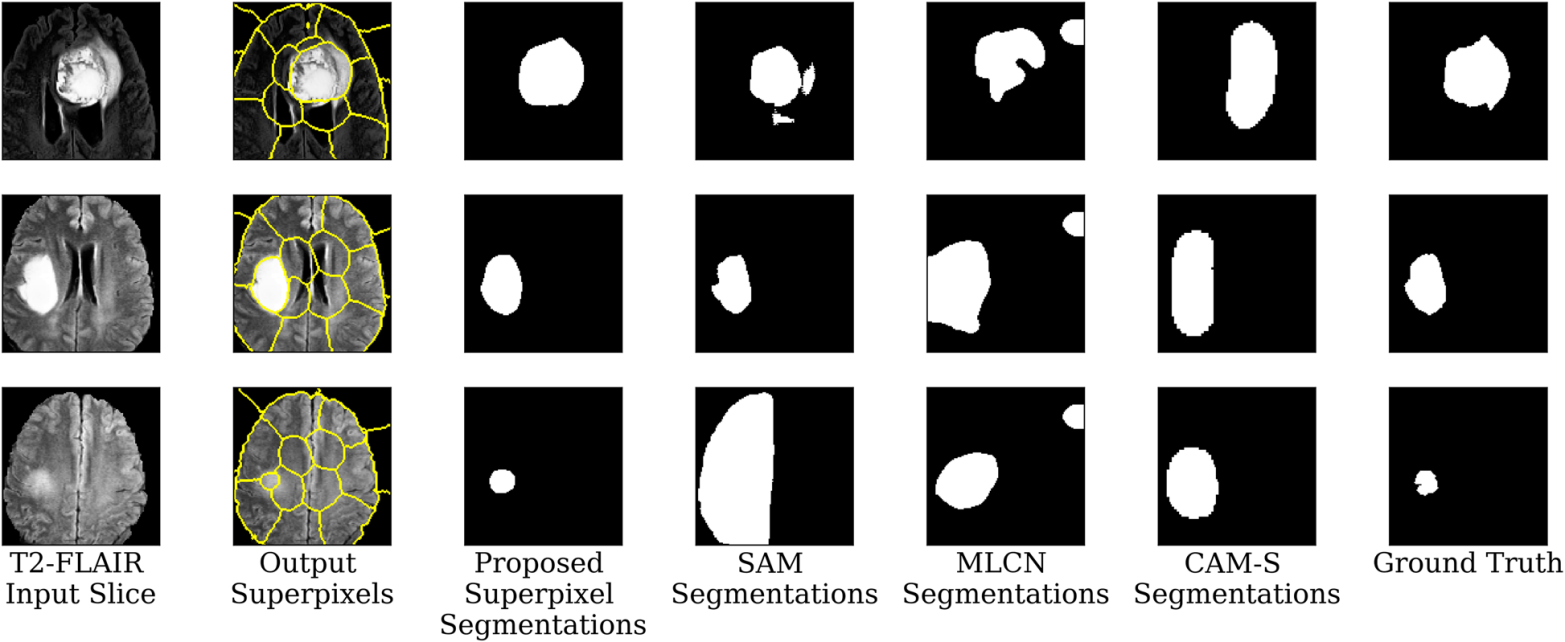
Visualization of T2-FLAIR channel of MR images, generated superpixels, output segmentations from the proposed method, output segmentations from the SAM, MLCN, and CAM-S baselines, and the true segmentations for three examples
